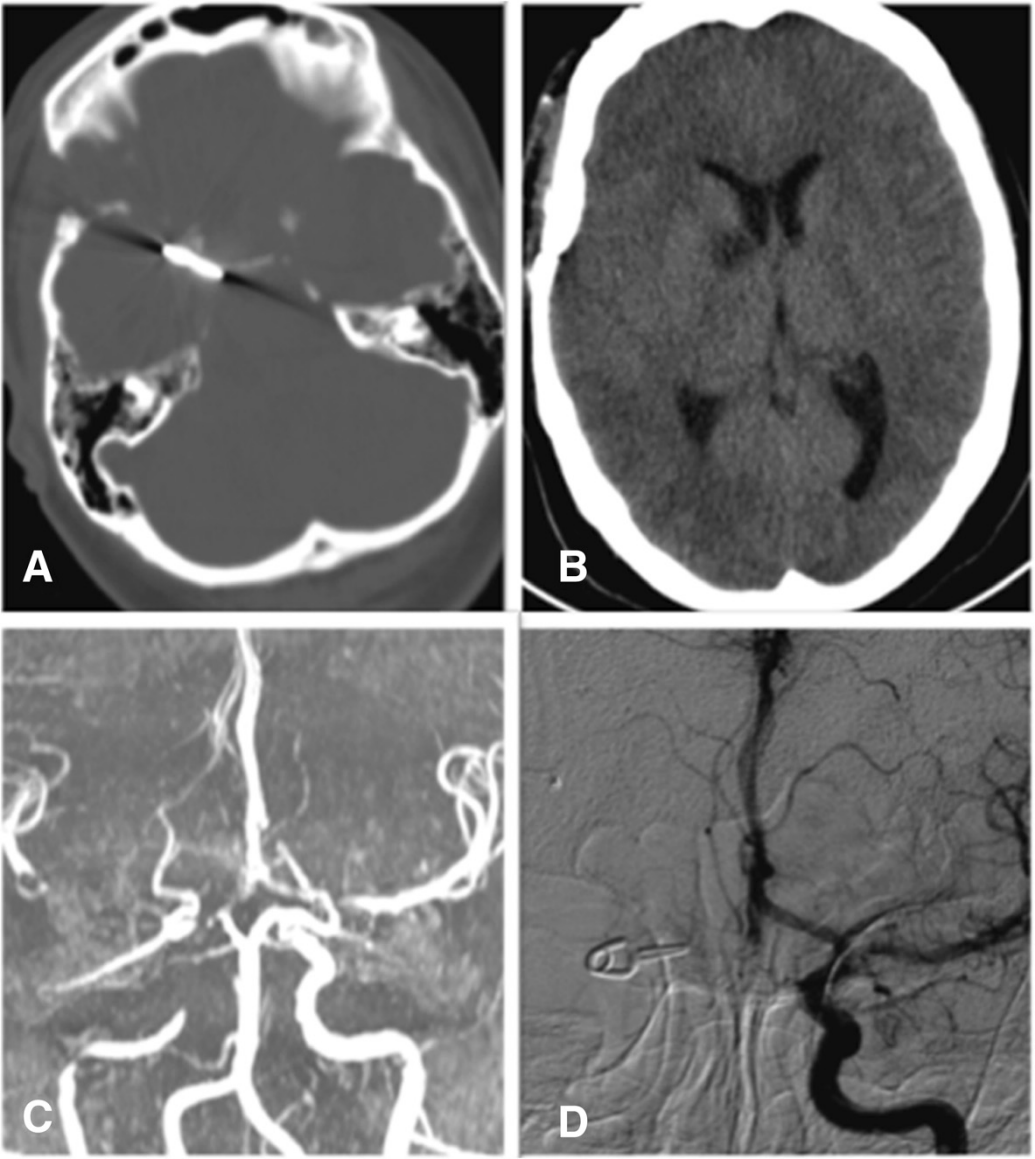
Computed tomography scans postoperative day 3 (A) showing the presence of the surgical clip at the level of the right anterior cerebral artery. Computed tomography scans postoperative day 7 (B) demonstrating right frontobasal hypodensity area – as per subacute ischemic stroke – and progressive resorption of the subarachnoid hemorrhage. Magnetic resonance angiography 1-month follow up (C) showing the regular perfusion of the areas normally supplied by the Circle of Willis. Three-months angiogram (D) highlighting the correct positioning of the clip, with regular perfusion of both right and left anterior postcommunicating cerebral arteries by the left carotid circulation.
